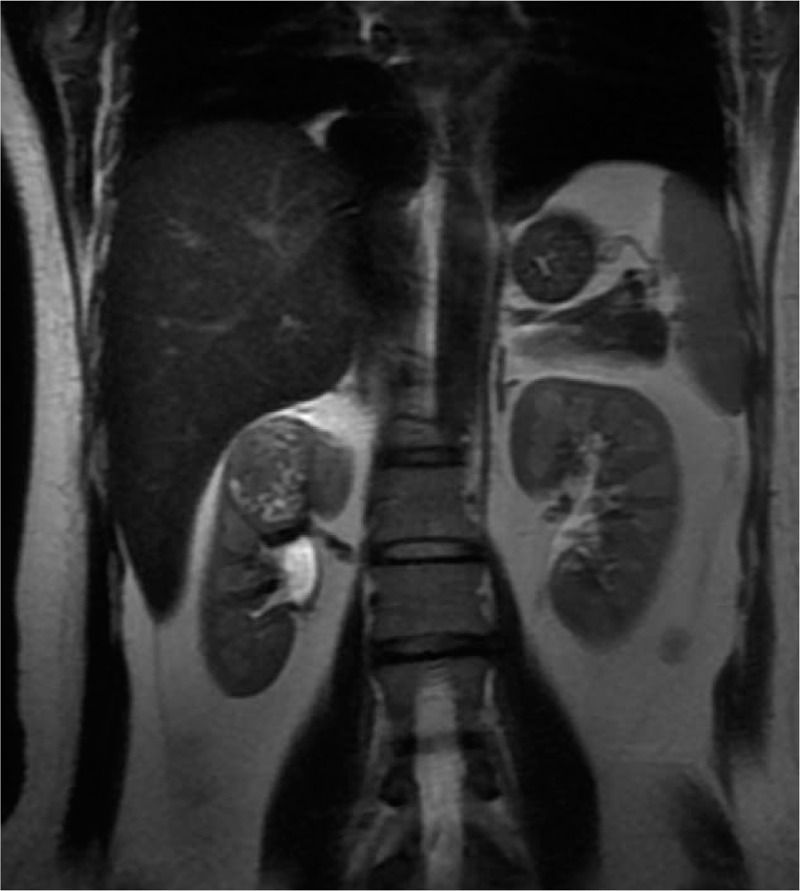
MRI demonstrating a lesion consistent with a primary renal neoplasm. MRI = magnetic resonance imaging.
